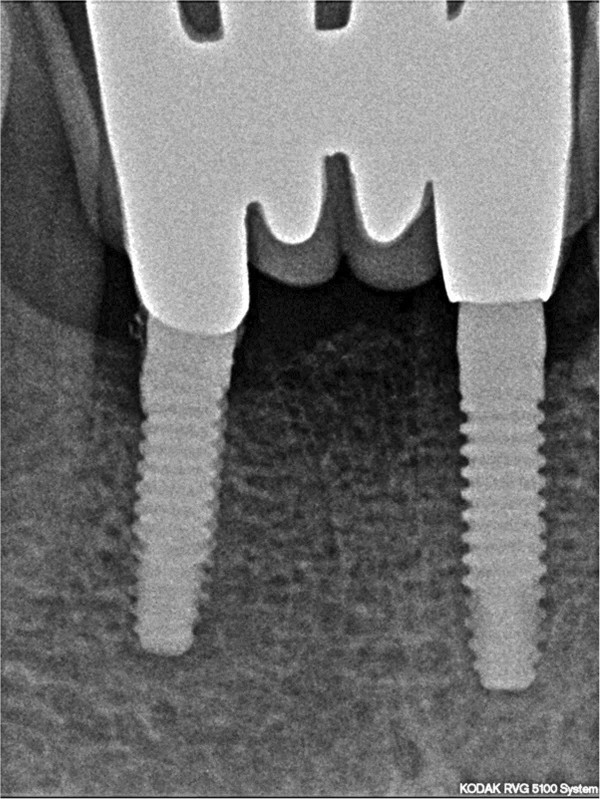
Panoramic X-ray after 1 year follow-up.
